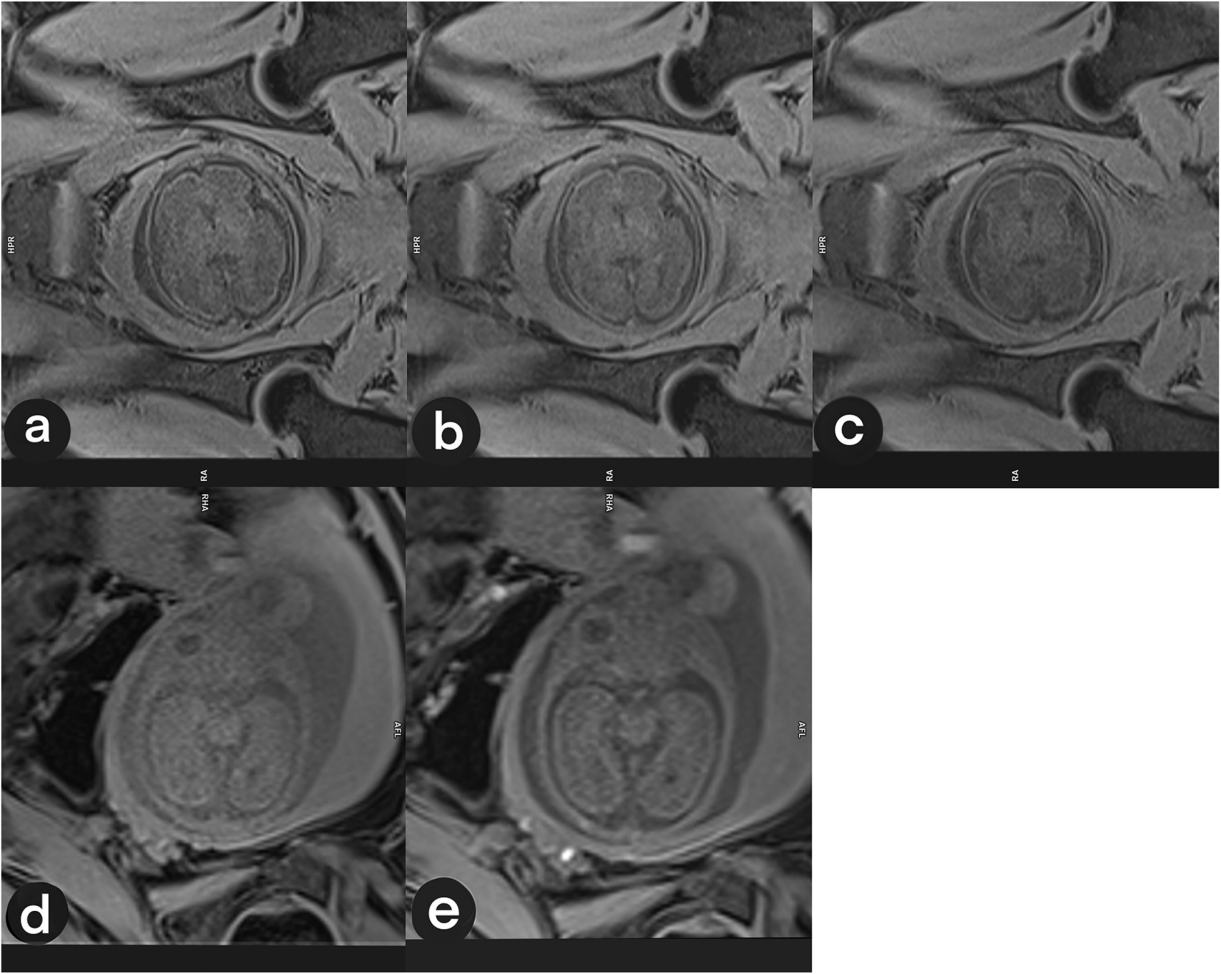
Transverse view of two fetal brains in different gestational ages, acquired by varying flip angle (FA) and repetition time (TR) with a T1-weighted 3D GRE fat-saturated sequence. a Repetition time = 4.1 ms and flip angle = 9°. b Repetition time = 6 ms and flip angle = 9°. c Repetition time = 7 ms and flip angle = 14°. d Repetition time = 4 ms and flip angle = 4°. e Repetition time = 6 ms and flip angle = 9°. A 29-week fetus is shown in the first row (a–c) and a 22-week fetus in the second row (d, e)
